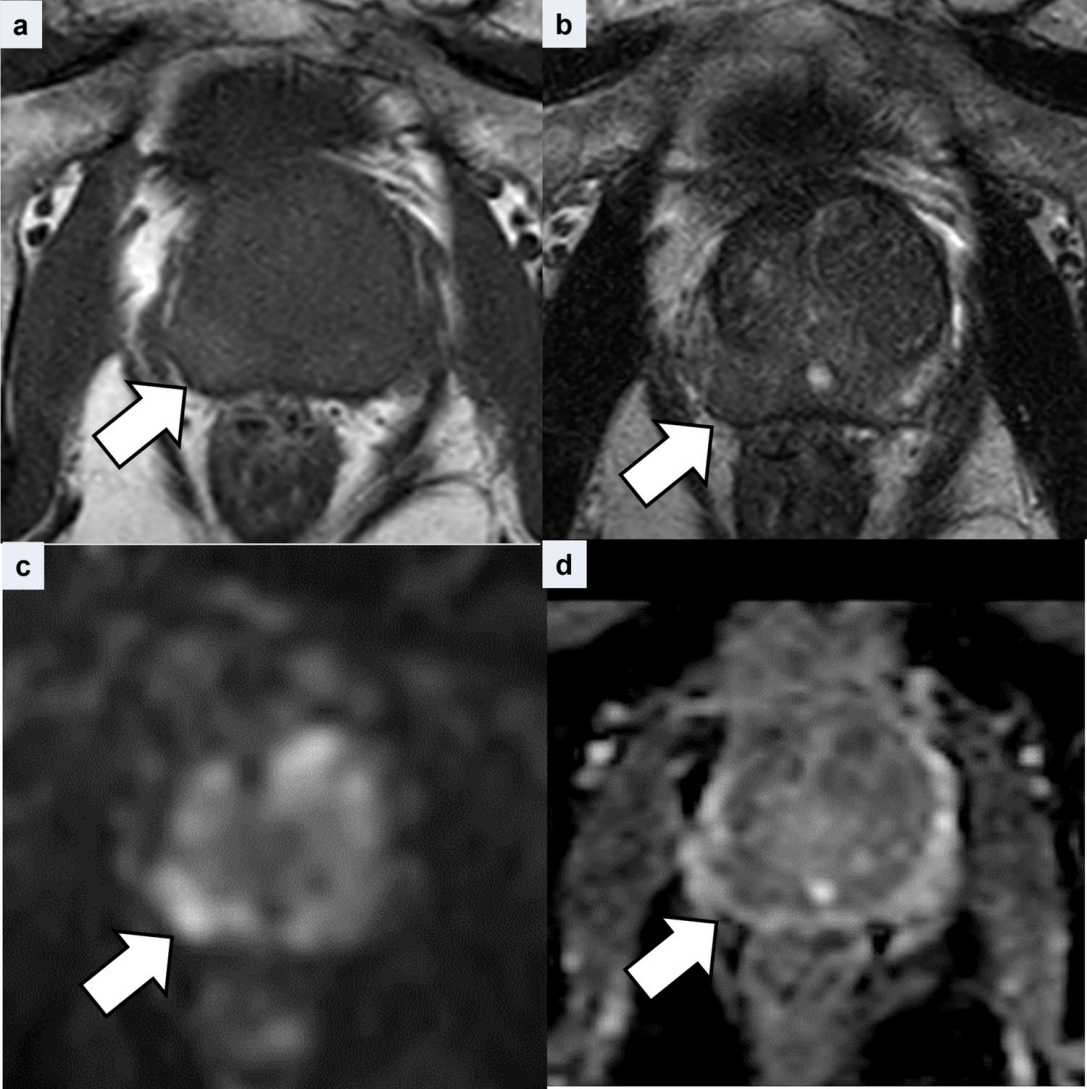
Representative case of diffuse lesion of granulomatous prostatitis in a man in his 70s. a Axial T1-weighted image shows diffuse hyperintensity in the right posterolateral peripheral zone (arrow). Lesion-muscle signal intensity ratio was 1.48. b On the corresponding T2-weighted image, the lesion demonstrates diffuse, moderate hypointensity (arrow). c The diffuse lesion demonstrates hyperintensity on axial diffusion-weighted image (arrow). d The corresponding ADC map shows decreased apparent diffusion coefficient values (0.73 × 10⁻^3^ mm^2^/s) (arrow)
